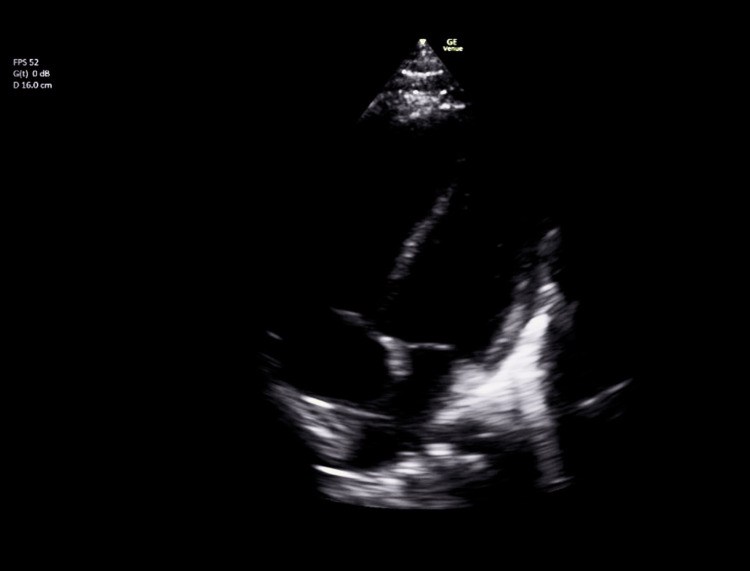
Point-of-care ultrasound demonstrating global hypokinesia
